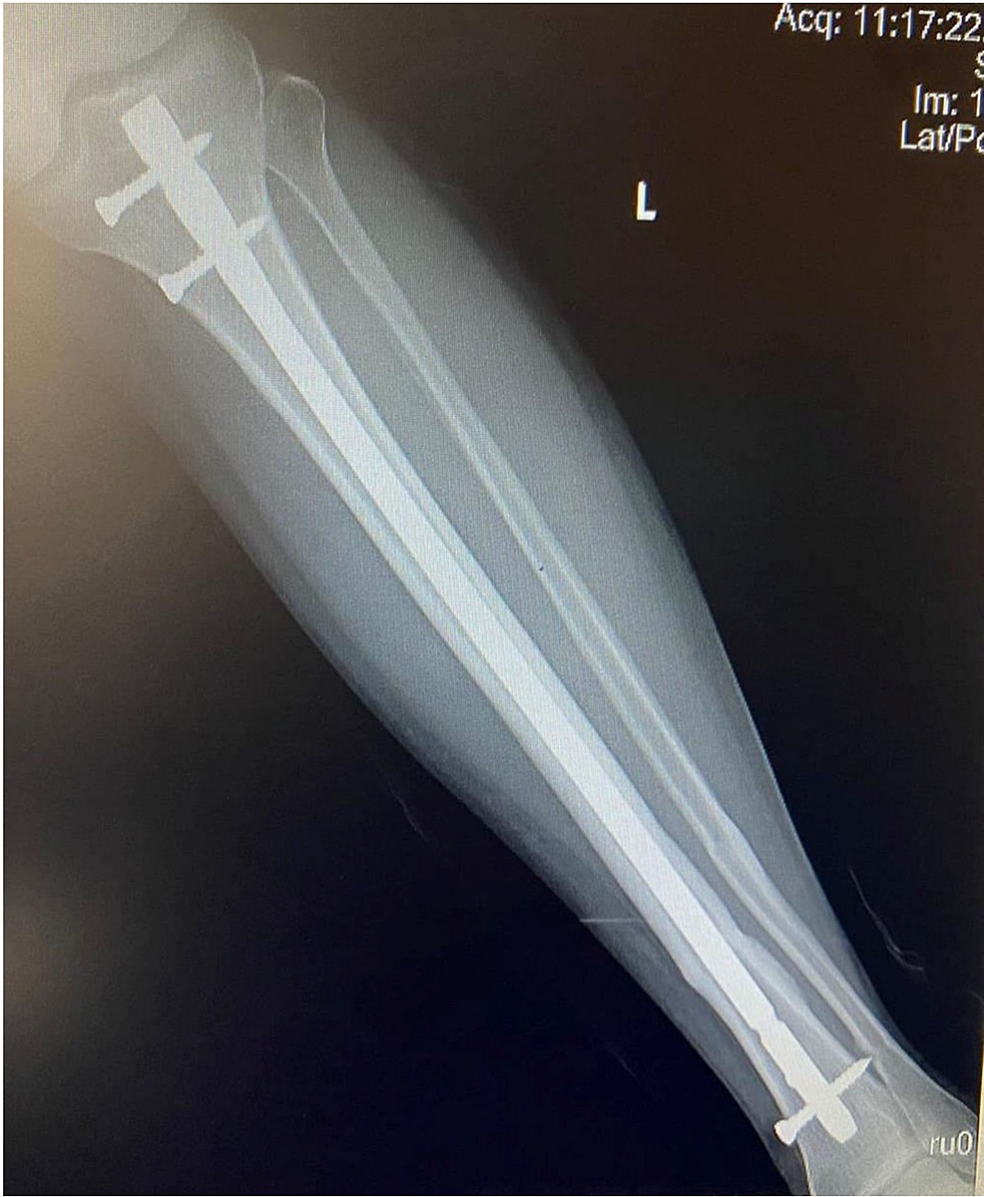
Six months follow-up AP radiograph of a 34-year-old nonsmoker showing united distal third fracture of the left tibia with IMIL nail in situ
